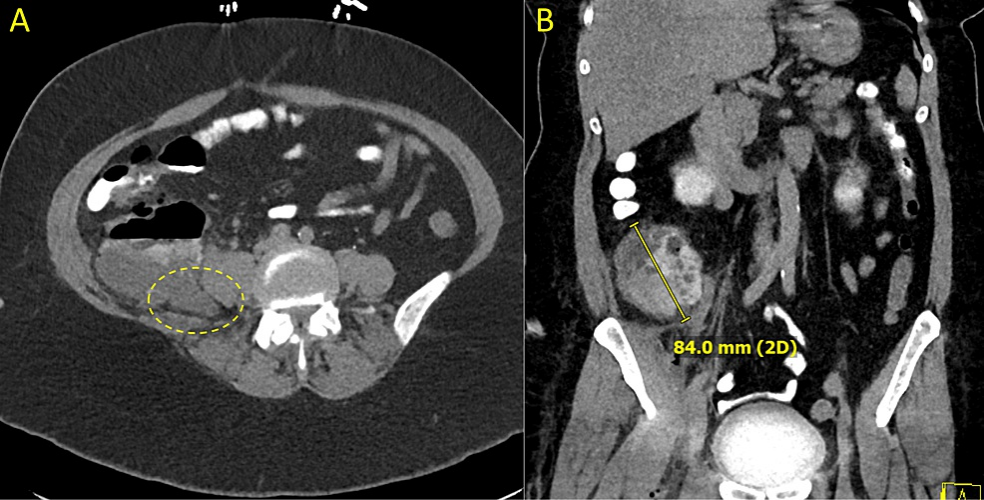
Computed tomography (CT) of the abdomen (A) Axial view demonstrating extensive inflammatory change consistent with ruptured retrocecal appendicitis (dotted circle). (B) Coronal view reveals a large intraluminal cecal abscess.
